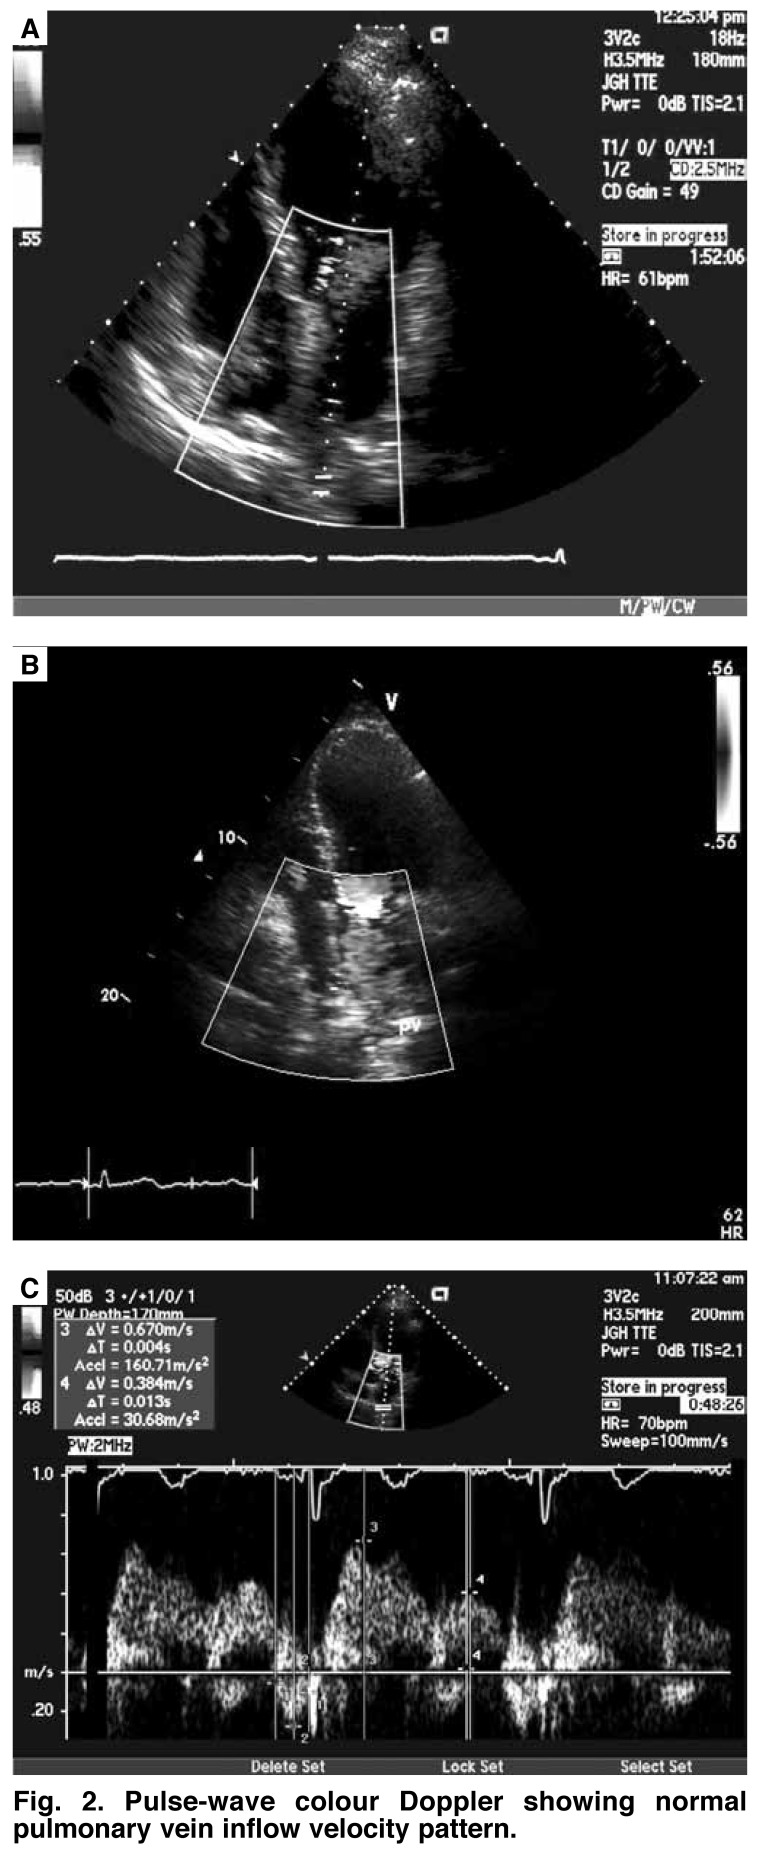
Pulse-wave colour Doppler showing normal pulmonary vein inflow velocity pattern.
